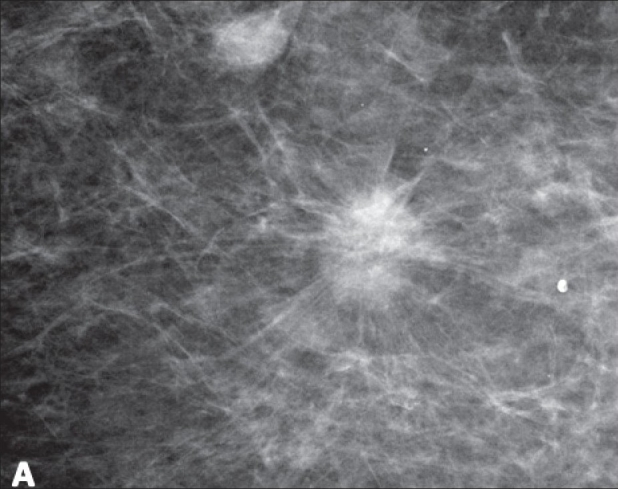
Screening mammography in a 67-year-old woman demonstrates a spiculated mass. Biopsy showed invasive ductal carcinoma, nuclear grade II. This was classified as BI-RADS 5Density descriptors include terms like high, equal, low, or fat-containing and are used to describe X-ray attenuation of the lesion relative to the expected attenuation of the surrounding fibroglandular breast tissue. For example, Figure 4 demonstrates a fat-containing lesion.
